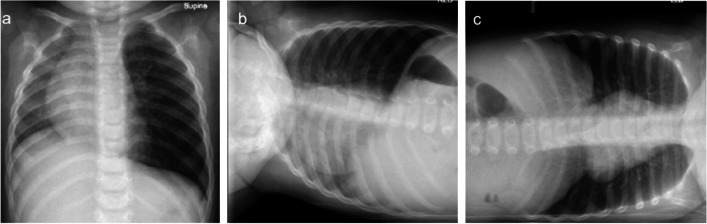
Radiographs of a 2-year-old boy who has choked whilst consuming carrots. a The anteroposterior (AP) view shows an expanded and hyperlucent left lung with a mediastinal shift to the right hemithorax. b Right lateral decubitus view shows a reduced aeration in the right lung, i.e. no sign of air trapping. c The left lateral decubitus view demonstrates the aeration in the left lung is maintained and the lung volume is almost constant to the contralateral side as well as unchanged compared to the AP view. The findings are conclusive with air trapping indicating a foreign body in the left main bronchus
